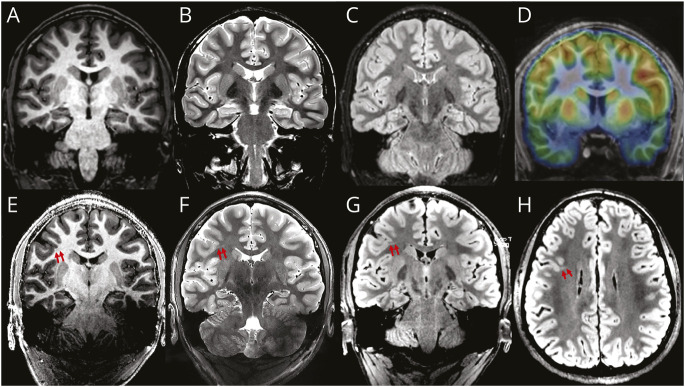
Case 3 (Patient 18) Patient affected by predominantly nocturnal seizures (up to 4/5 times per week). The video-EEG captured seizures with electrical correlate from the right frontal region; however, the 3T MRI (top row; A: MPRAGE; B: T2w; C: FLAIR) was reported as normal. The PET scan (D) detected a very focal reduced tracer uptake in the right inferior frontal sulcus. The 7T MRI (bottom row; E: MP2RAGE; F: T2w; G, H: FLAIR) highlighted a faint band of mildly increased signal in the FLAIR image extending into the white matter from the deep cortical margin in the depth of the right inferior frontal sulcus posteriorly (transmantle sign). This was detected precisely in the same sulcus as seen in the PET-MRI co-registration. The stereo-EEG confirmed epileptic activity originating from that area. A thermocoagulation procedure was undertaken, and the patient was seizure free at 2 years of follow-up. FCD = focal cortical dysplasia; FLAIR = fluid-attenuated inversion recovery; MPRAGE = magnetization-prepared rapid gradient-echo; MP2RAGE = magnetization-prepared 2 rapid acquisition gradient-echo; T2w = T2-weighted.
